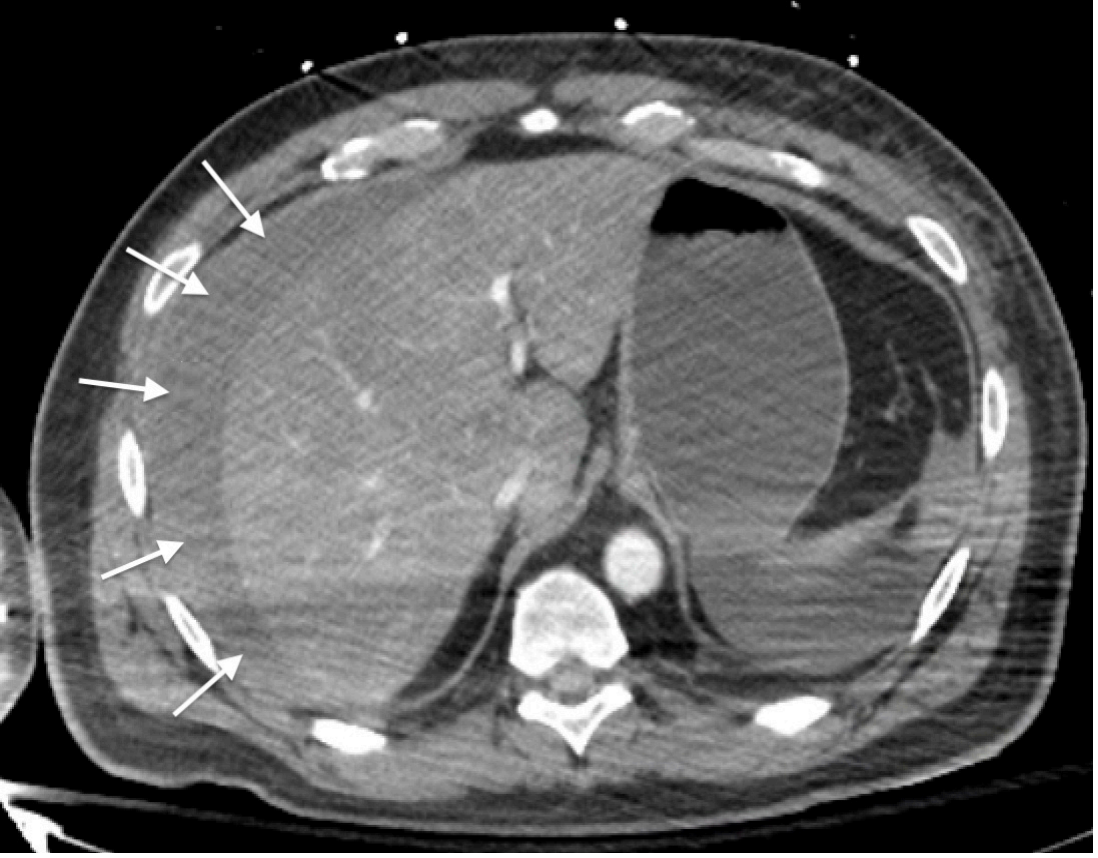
Computed tomography of abdomen and pelvis with contrast showing subscapular hyperdense blush along the right lower hepatic lobe. Favored active venous subscapular bleeding of liver with new hemoperitoneum from intraparenchymal hematoma given timing of contrast phase. Subcapsular hematoma is visible around the liver (arrows).
